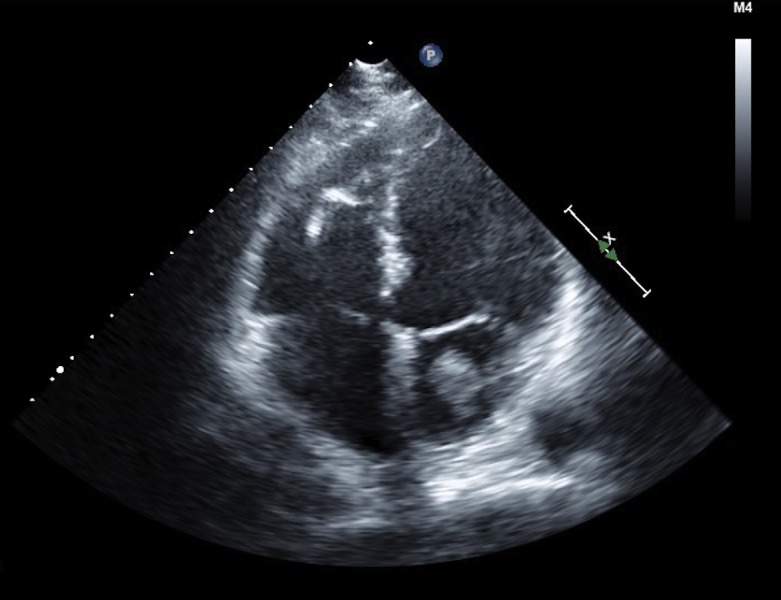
Transthoracic echocardiogram, 4-chamber view, demonstrates a left atrial mass measuring up to 5 cm in length.
